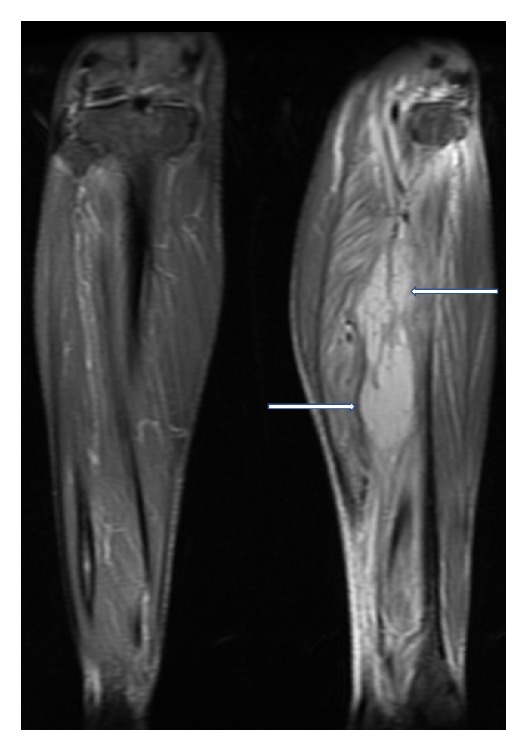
Coronal, T2 weighted, fat saturated, STIR MR image showing loculated fluid signal within the tibialis posterior muscle (thick white arrows), suggestive of a collection. Also noted are diffuse T2 hyperintense signal changes in the surrounding musculature (thin white arrows), compatible with myositis.
